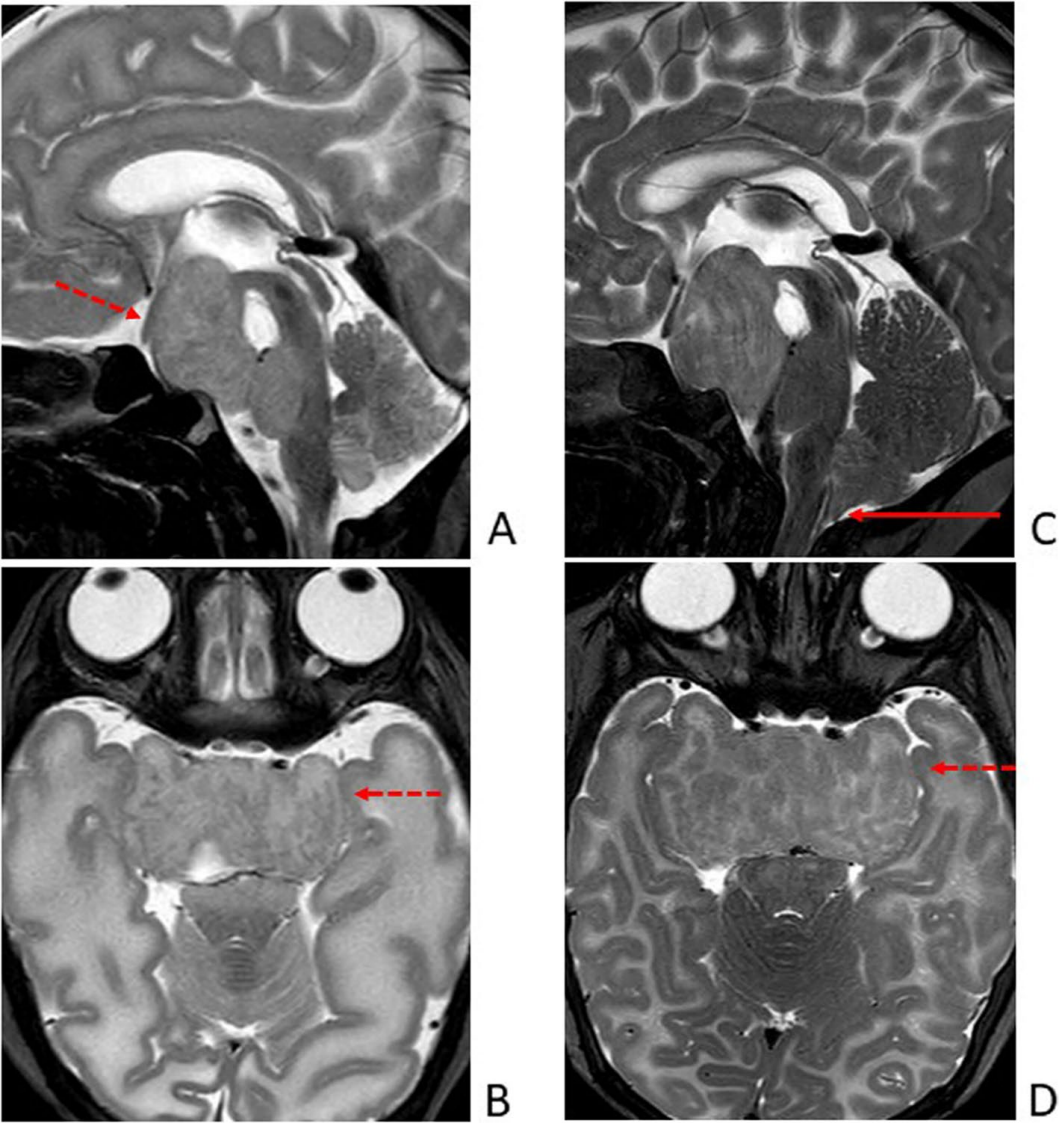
Brain MRI of Twin B comparing TSE T2 sagittal (A) and axial (B) images performed at 35^+ 6^ weeks' gestation, with the same images (C, D) performed at 50^+ 6^ weeks of corrected age: the lesion (dotted red arrow) increased in size causing a more relevant mass effect on the surrounding structures and an initial displacement of the cerebellar tonsils through the foramen magnum (red arrow). The lesion still appeared isointense to the surrounding parenchyma, with myelination-like aspects and thickening of the intra-lesional cortical component
